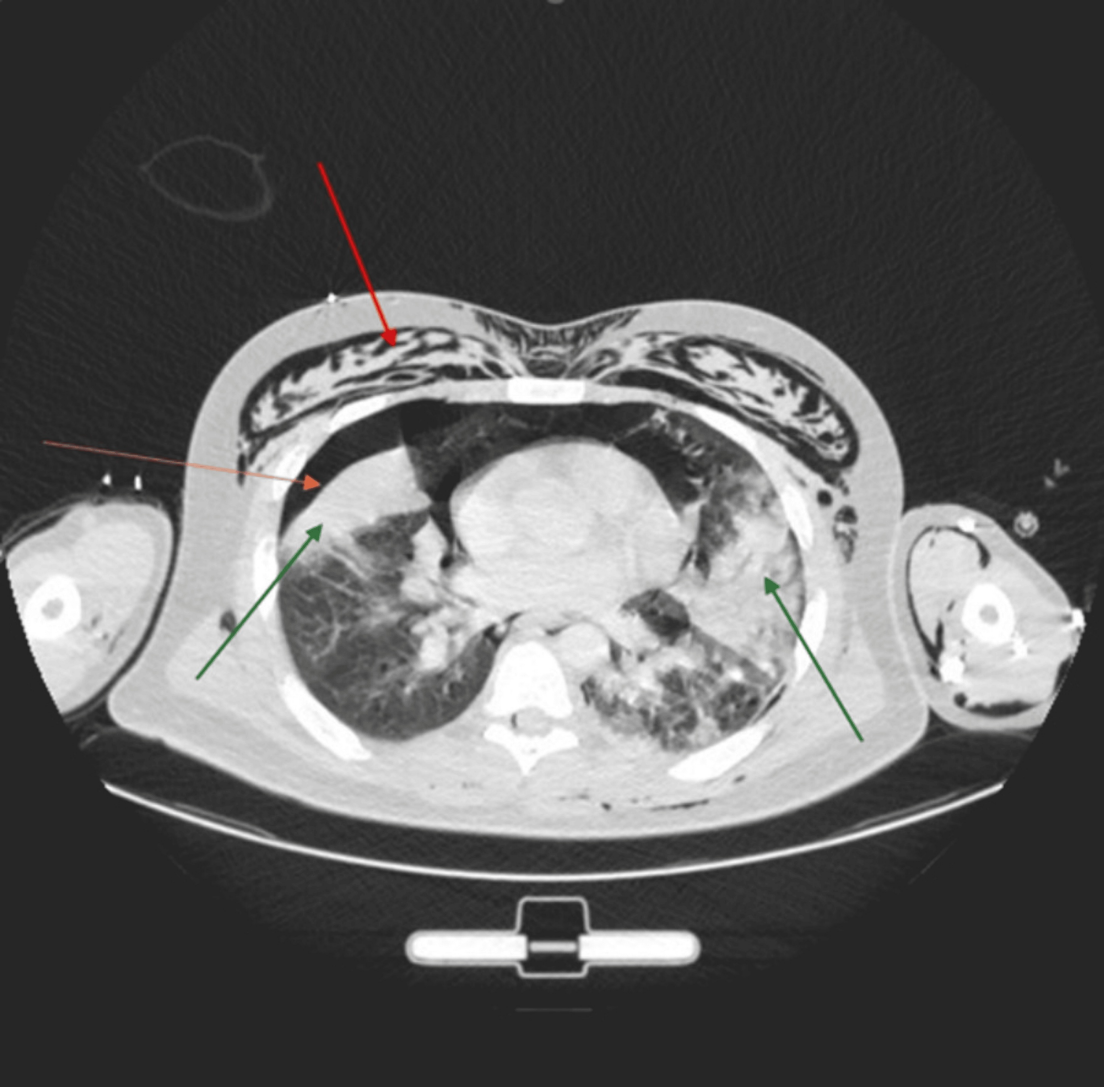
CT scan showing significant subcutaneous emphysema (red arrow), right-sided pneumothorax (orange arrow), and bilateral consolidation (green arrows).
